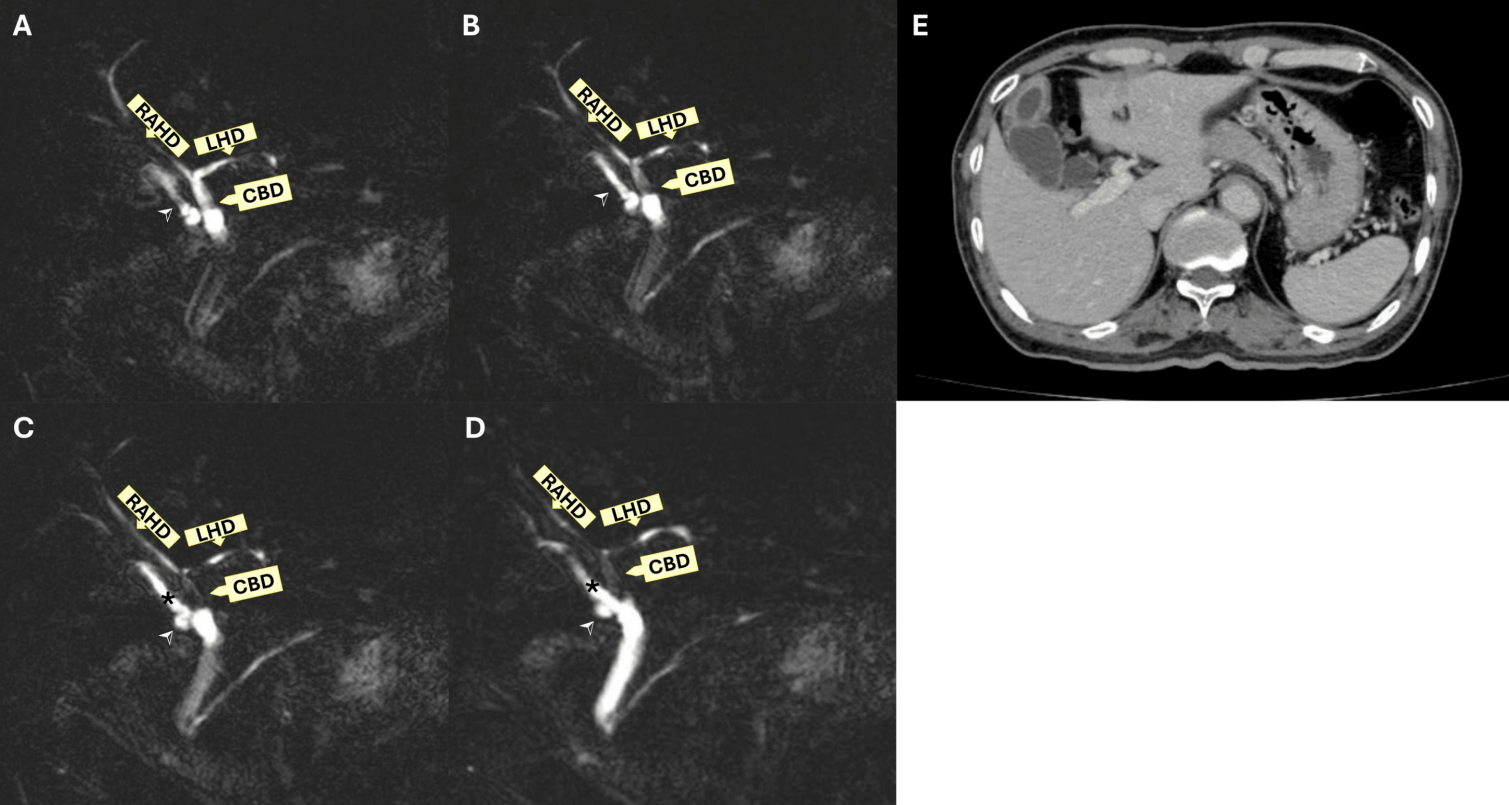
Preoperative images showing a variant of the right posterior hepatic duct A, B, C, D: Magnetic resonance cholangiopancreatography (MRCP) show the cystic duct (arrowhead) confluent to the variant of the RPHD (asterisk) that drains directly into the common bile duct (CBD). E: Axial CT scan demonstrates localized mural thickening of the gallbladder wall, predominantly at the fundus and body. There is no evidence of pericholecystic fat stranding. Both intrahepatic and extrahepatic bile ducts are not dilated. RPHD: right posterior hepatic duct; RAHD: right anterior hepatic duct; LHD: left hepatic duct
